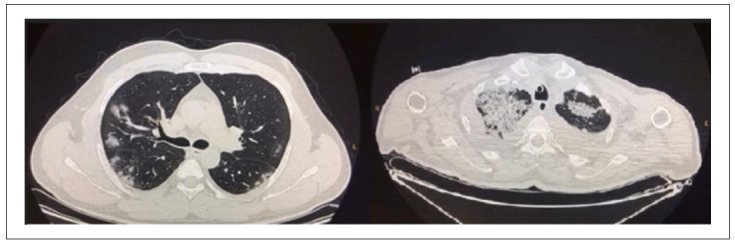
Computed tomography (CT) sample images of patients with COVID-19.
